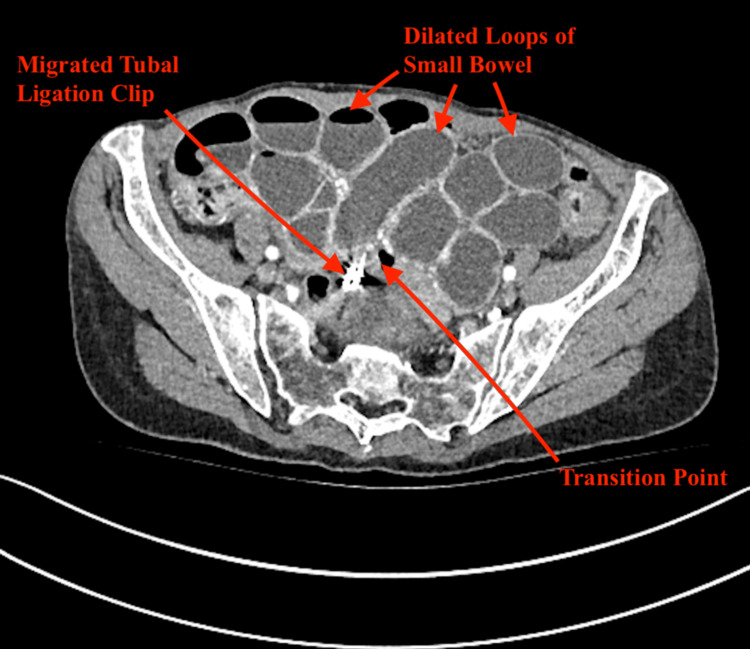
Contrast-enhanced CT of the abdomen demonstrating multiple dilated small bowel loops. The transition point is closely associated with a migrated tubal ligation clip.
